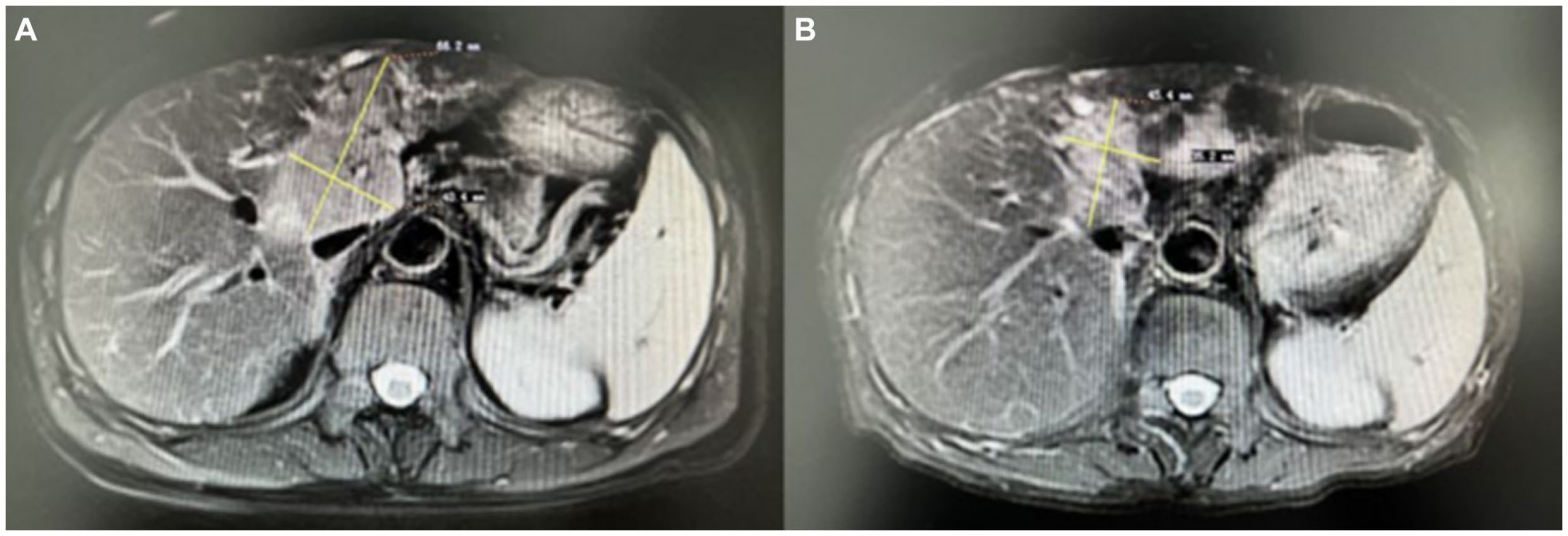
Liver MRI with gadolinium contrast showed the presence of a malignant tumor before treatment (A), and after treatment, the size of the liver tumor was reduced (B).
